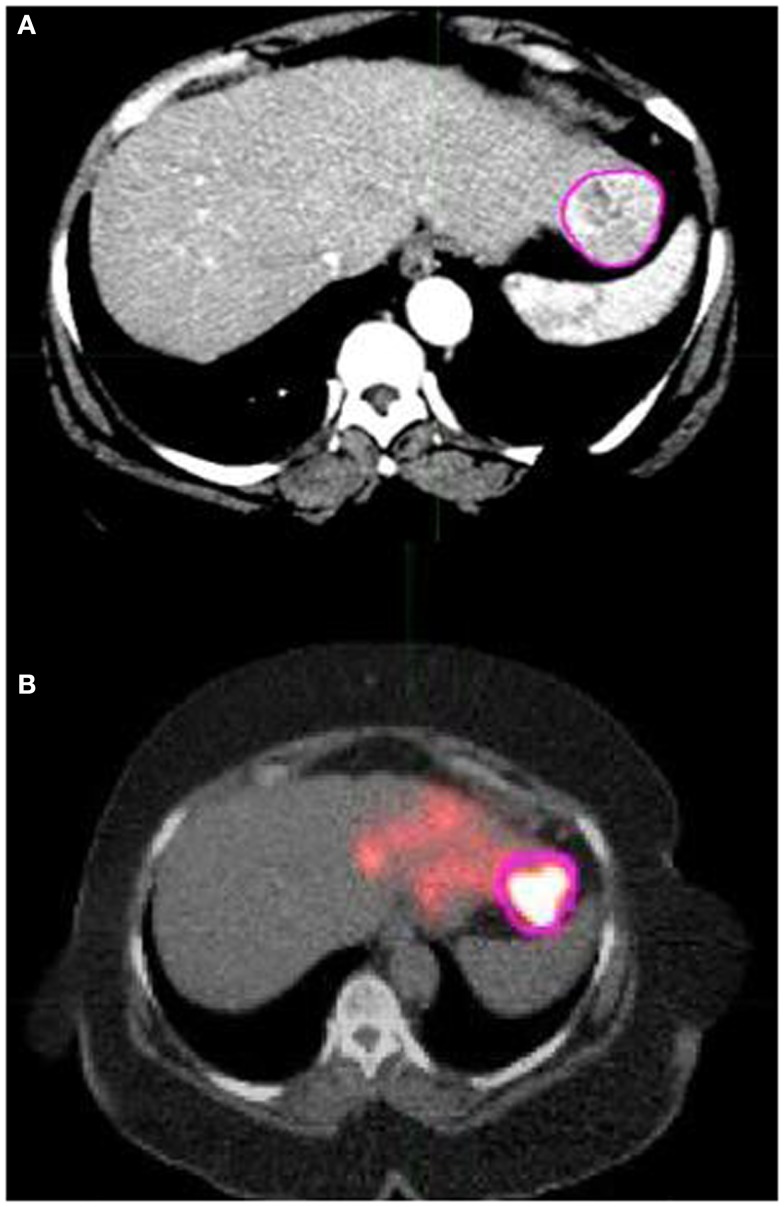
(A) shows a pink contour drawn around the tumor in an axial slice of the deformed version of the arterial phase CT scan that was shown in Figure 2A. (B) shows the same tumor contour overlaid on the same AC CT/PET fusion scan shown in Figure 2B that was acquired following left lobe ^90^Y microsphere therapy. (B) exhibits that contours drawn on the deformed arterial CT show good overlap with the corresponding region of interest in the AC CT/PET fusion image. Because this allows for more accurate contour statistic calculations, the deformation tools in MIM were utilized.
